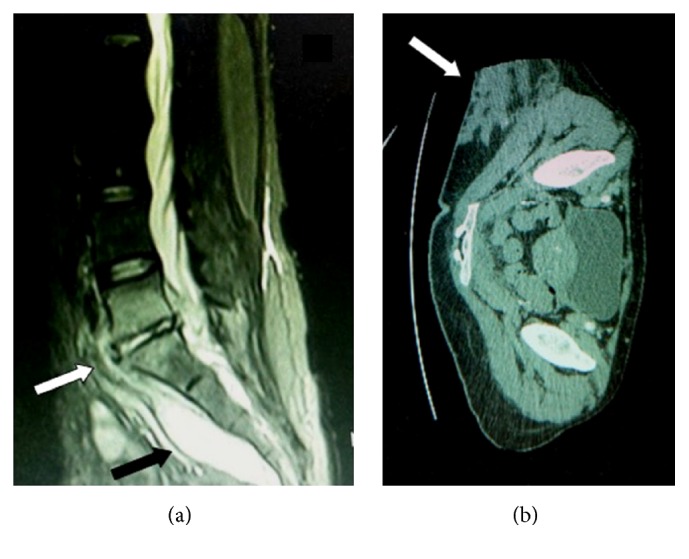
(a) Presacral epidural inflammatory fluid collection (abscess) (black arrow) and adjacent spondylodiscitis that developed at the level of L5-S1 vertebrae (white arrow). Due to its small size the epidural abscess was successfully managed with antibiotics alone. (b) Large abscess of the right buttock (white arrow) that was drained under CT scan guidance.
